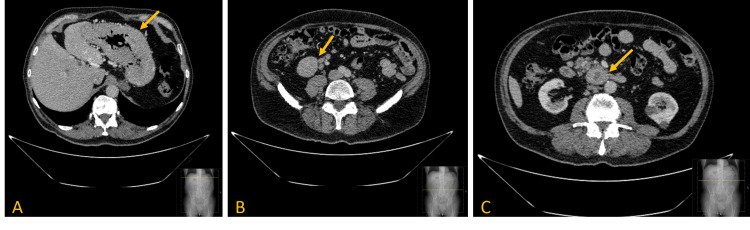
Cross-sectional CT images of the abdomen and pelvis obtained in October 2021 Image A: Cross-sectional CT image of the abdomen and pelvis demonstrating gastric wall thickening (yellow arrow). Image B: Cross-sectional CT image of the abdomen and pelvis demonstrating multiple pathological lymph nodes (yellow arrow). Image C: Cross-sectional CT image of the abdomen and pelvis demonstrating a possible duodenal mass (yellow arrow).
